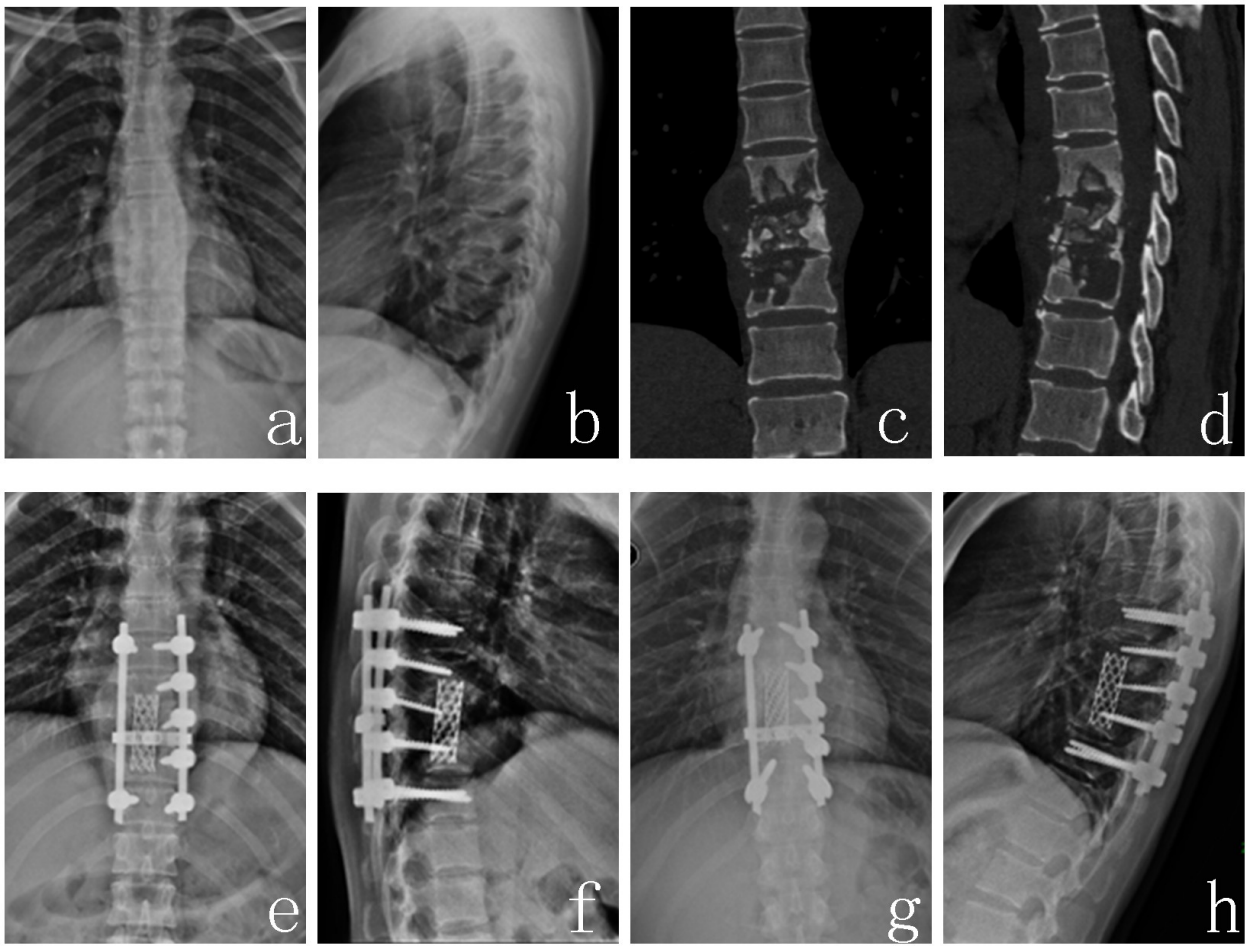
A 41-year-old female patient with T8,9,10 spinal tuberculosis (Frankel grade E) got formed titanium mesh for reconstruction. (a~d) Preoperative X-ray and CT demonstrated that T8-10 vertebra body and the intervertebral discs were destroyed and instability was formed. (e, f) Postoperative X-ray, (g, h) A-P and lateral X-ray at 31 months after surgery revealed good location of titanium mesh and lumbar physiological curve.
